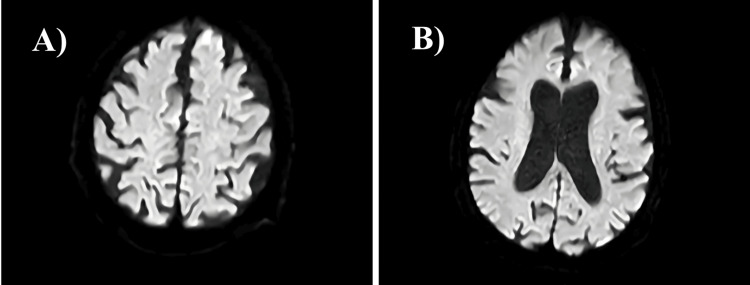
Images A and B show axial magnetic resonance imaging (MRI) scans from an examination of an 82-year-old male with no acute abnormalities, demonstrating age-related involutional changes.
